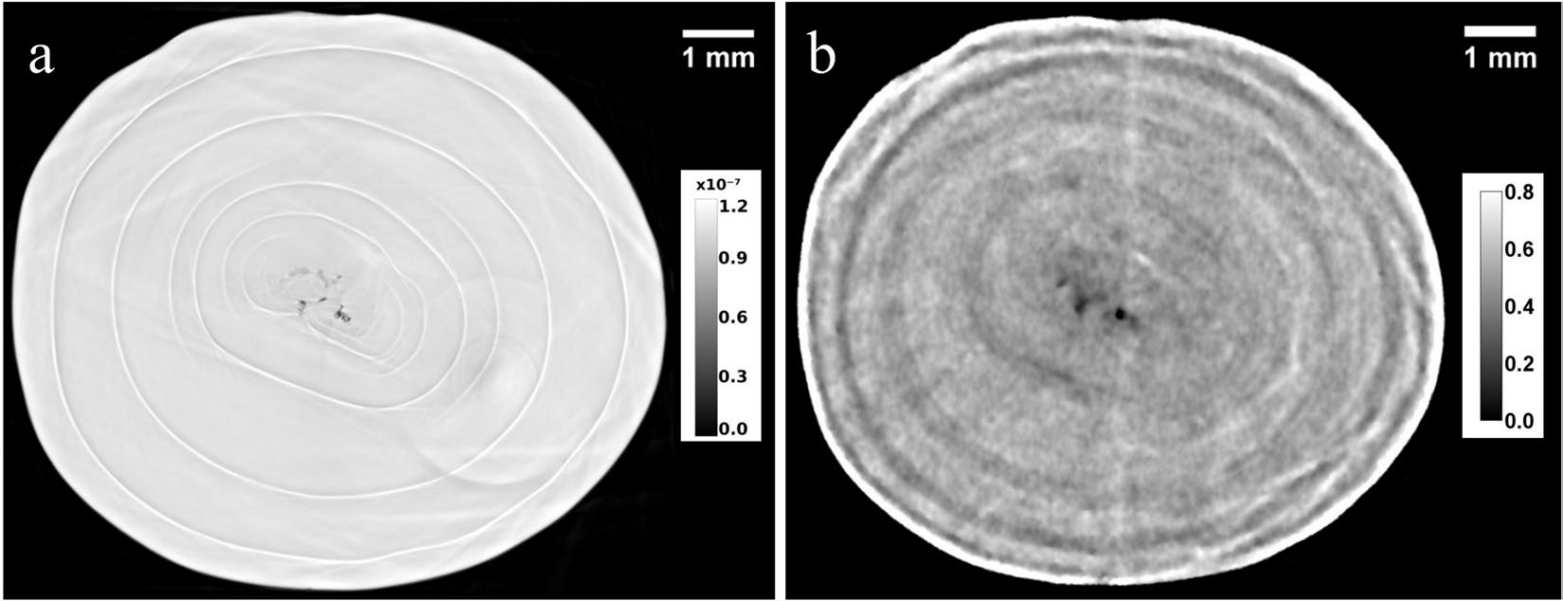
X-ray PC (a) and neutron (b) CT reconstructed images corresponding to the same slice of the cultured freshwater pearl CP-21. Several growth rings and small voids within the pearl are visible in both reconstructed images. Grey scale refers to refractive index decrement for PCI (a) and to linear attenuation coefficient (cm^−1^) for NI (b).
